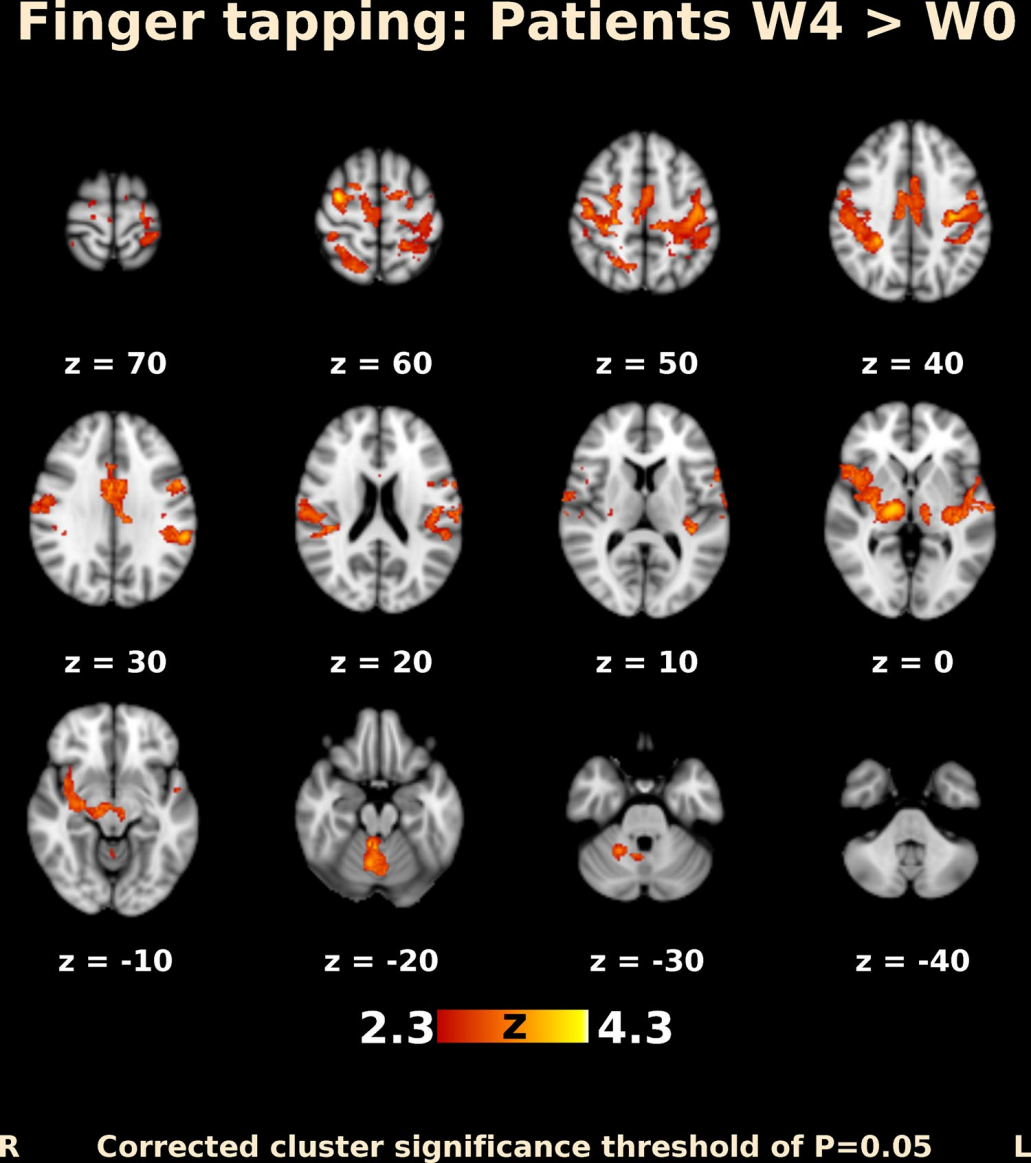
Functional MRI activation map (transversal slices) in patients with CD. Differences in activation after and before BoNT-A injection. Slices are labeled with *Z*/*Y* coordinate in standard MNI152 space
